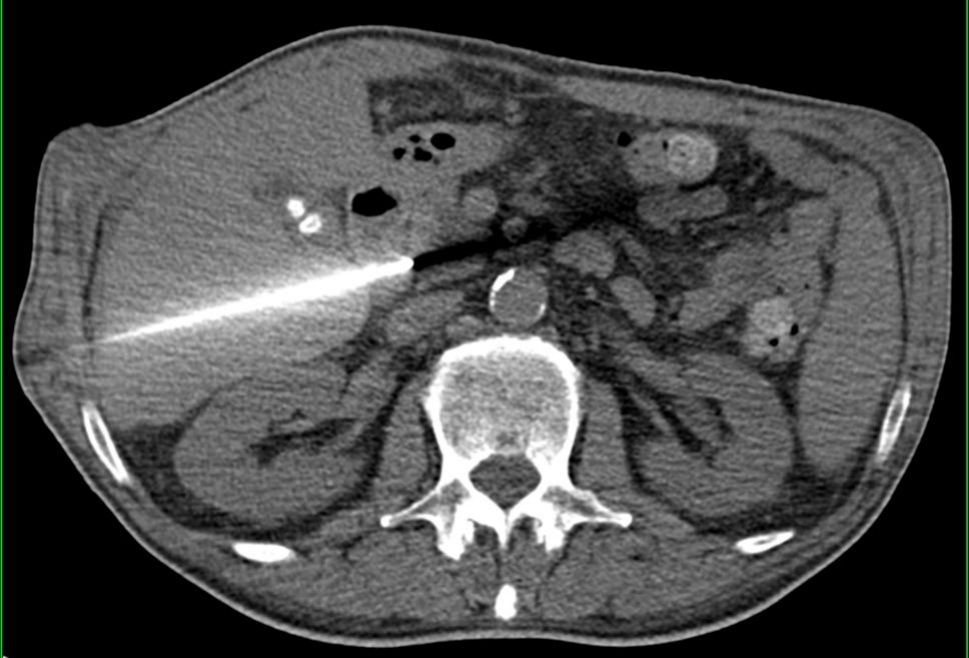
CT-guided transhepatic biopsy of a lesion in the pancreatic head. The needle trajectory passes through the liver and duodenum toward the target lesion
